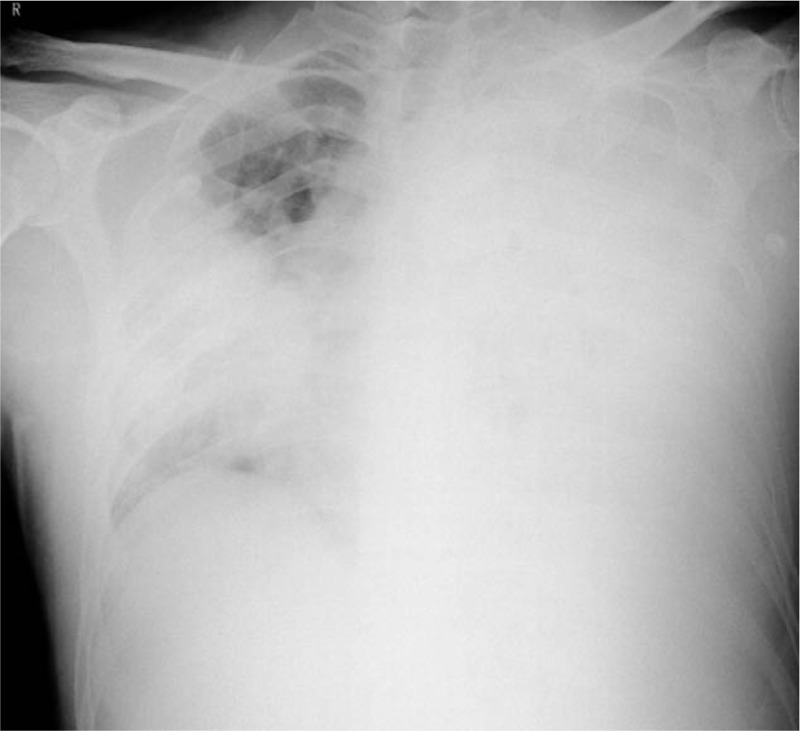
Chest X-ray of the male patient after 1 week of treatment revealed consolidations on bilateral lungs. CT = computed tomography.
